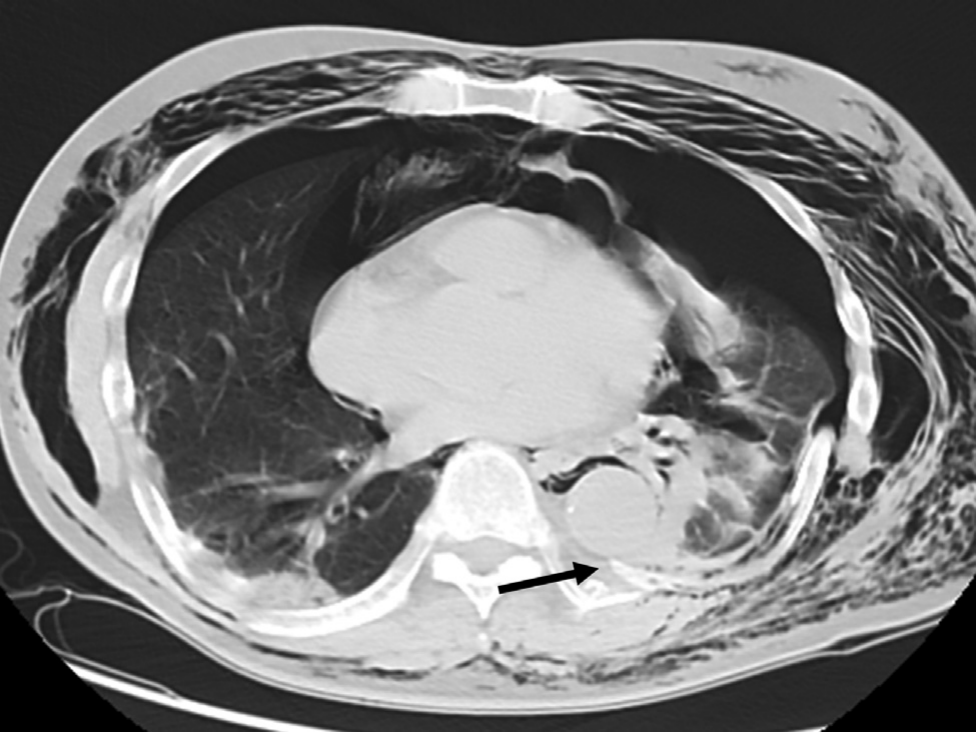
Thoracic computed tomography (CT) on arrival in case 1. CT shows the spiculated end of the ninth fractured rib adjacent to the descending aorta (arrow).
